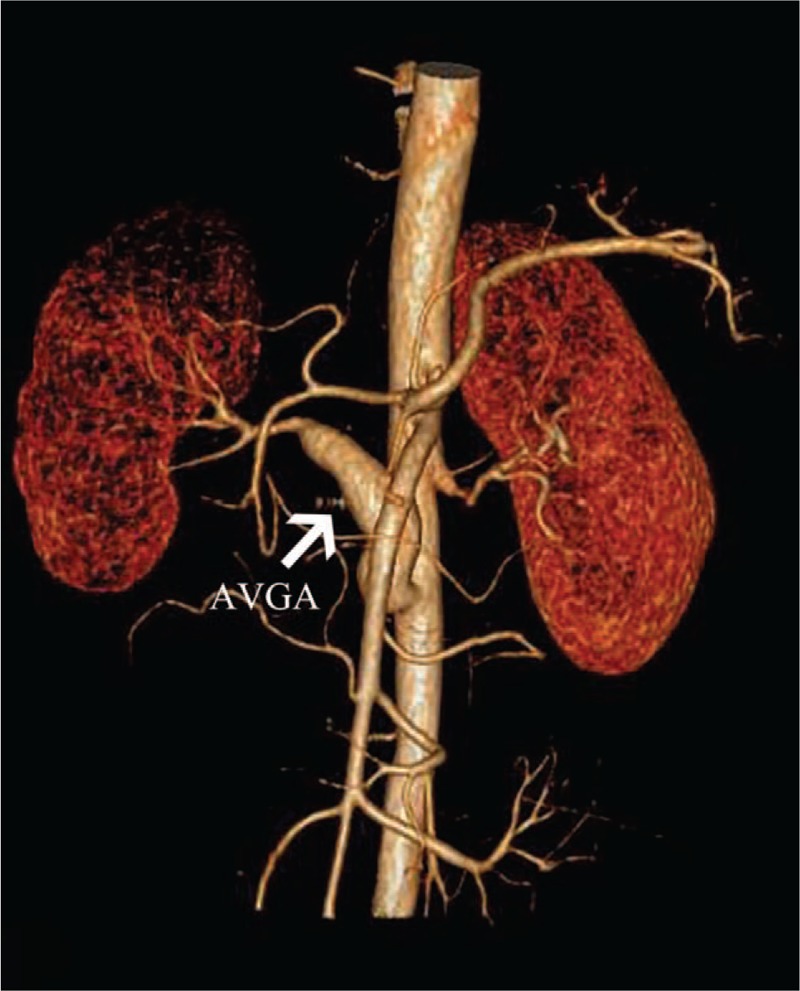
Two years after aortorenal bypass with SVG. The updated CTA revealed AVGA with a maximum diameter of 2.6 cm.
